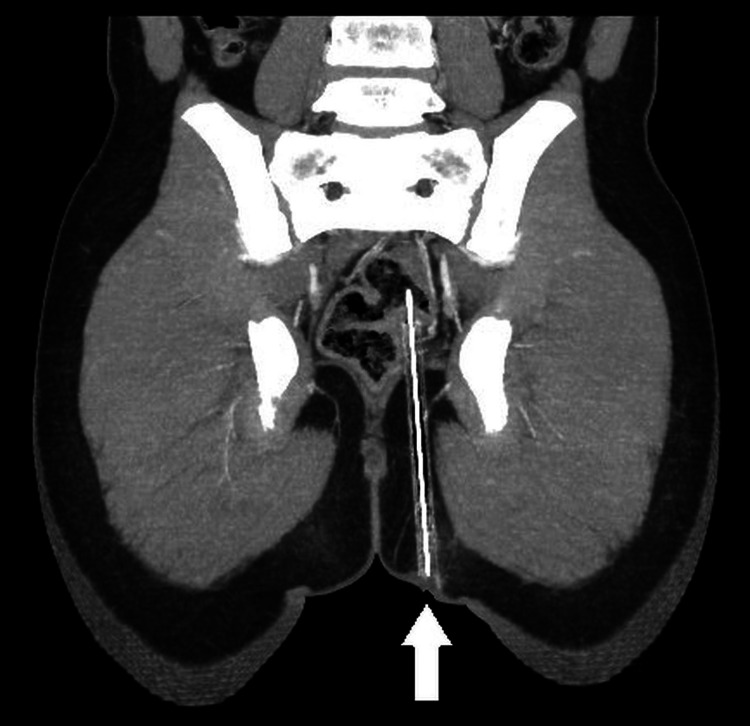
Coronal CT scan shows a hyperdense linear foreign body (white arrow) extending from the inferomedial left gluteal region into the pelvis. The tip is seen approaching the posterior wall of the rectosigmoid junction, with no signs of free air or fluid.
